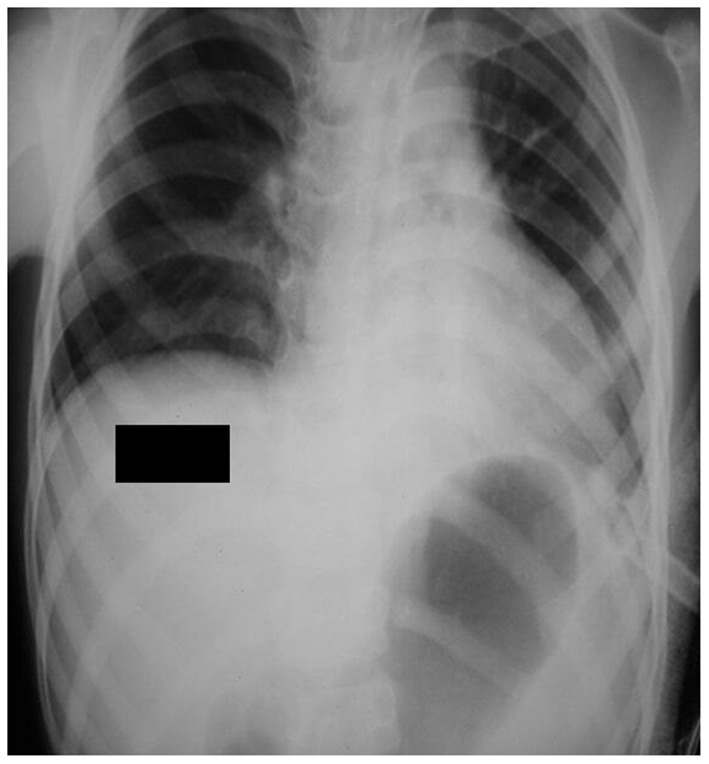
The follow-up chest x-ray shows a normal mediastinum with the gastric bubble present below the diaphragm.
